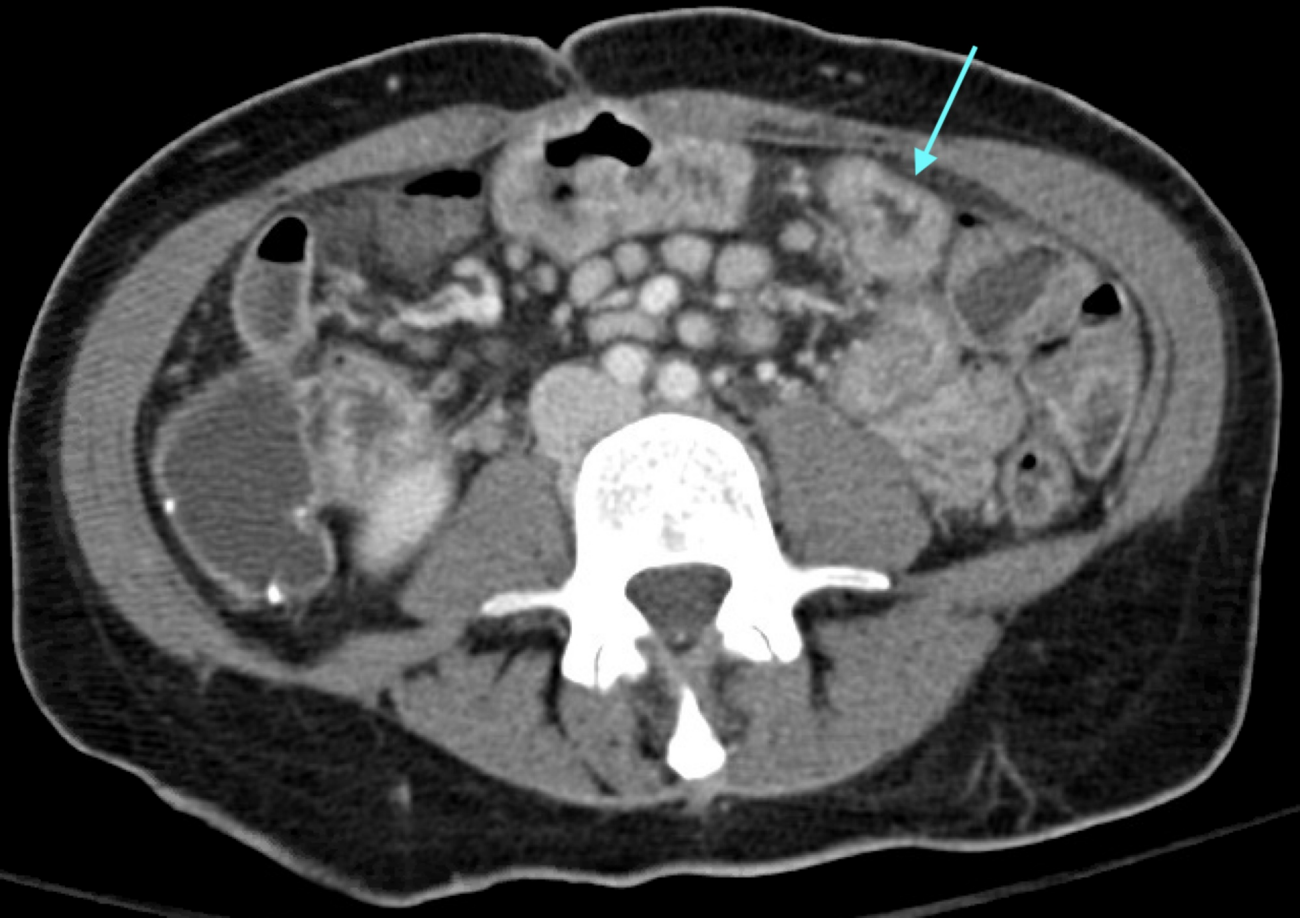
CT abdomen and pelvis with IV contrast The blue arrow points to the region of small bowel wall edema.
